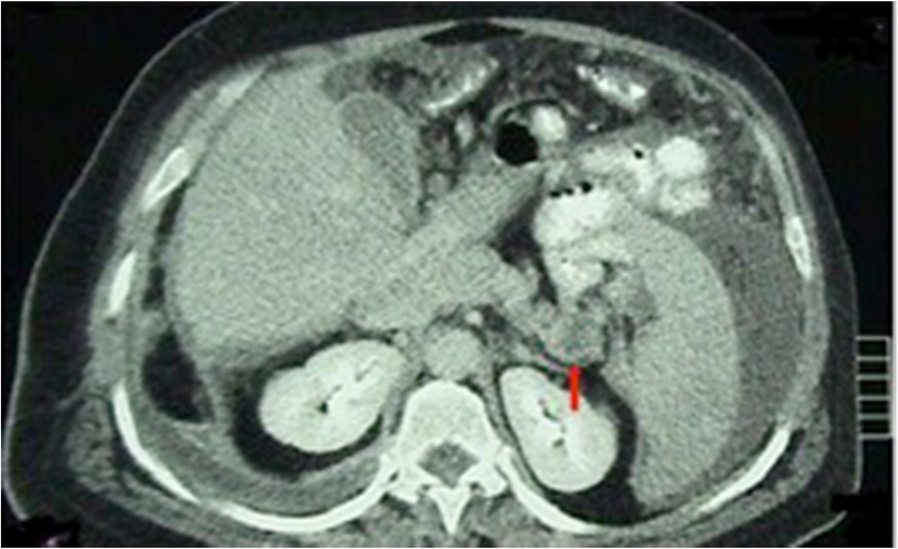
Computed tomographic scan. Densitometric alteration of the tail of the pancreas can be seen (inhomogeneous hypodensity, red arrow)
